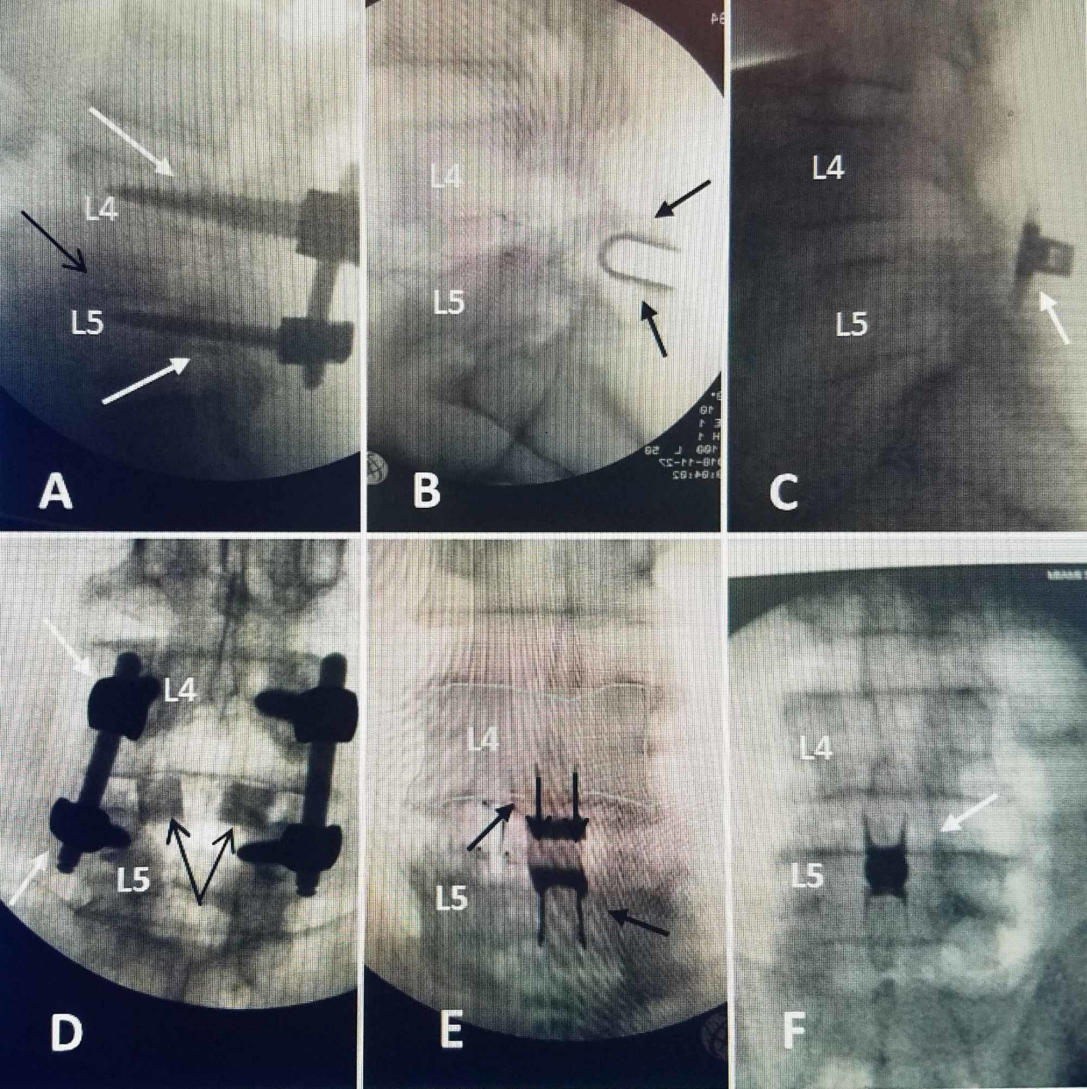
Postoperative radiographs of different L4-5 stenosis cases showing relative size difference between pedicle screws, Coflex® interlaminar and Vertiflex® interspinous implants. The photographs were kept to similar size so the size of the different systems can be compared. A: Lateral postoperative radiograph of one level L4-5 pedicle screw fixation (solid white arrows) with bilateral decompression, medial facetectomy and foraminotomy with interbody grafts (solid black arrow). B: Lateral radiograph of one-level Coflex® implant (solid black arrows) at L4-5. C: Lateral radiograph after L4-5 one-level Veriflex® implant (solid white arrow). D: Anterior-posterior radiograph showing L4 and L5 pedicle screws and rods and two interbody implants in the L4-5 interspace (solid black arrows). E: Anterior-posterior view of L4-5 Coflex® in after interlaminar decompression positioned between the two spinous processes at L4-5 (solid black arrow). F: Anterior-posterior view of Vertiflex® interspinous device (solid white arrow) placed percutaneously.
